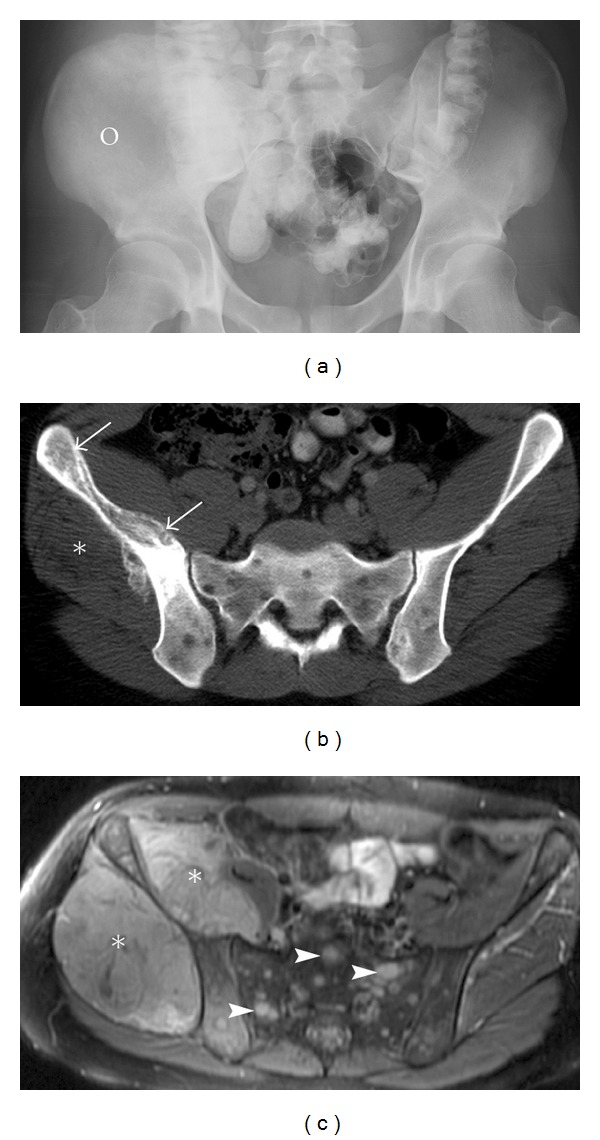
Ewing's sarcoma. A 21-year-old male with advanced Ewing's sarcoma. AP radiograph (a) of the pelvis reveals a vague mixed density abnormality diffusely involving the right ilium (circle). Note the asymmetry when compared to the normal left ilium. Incidental note is made of oral contrast in the bowel. CT scan (b) of the pelvis demonstrates permeative lesion of the right ilium (arrows) and an associated soft tissue mass (asterisk). Small multifocal lytic lesions in the sacrum and bilateral ilium are in keeping with metastasis. Axial T2 image (c) of the pelvis reveals a large exophytic soft tissue mass (asterisk) along the anterior and posterior borders of ilium. Note the predominance of a huge soft tissue mass when compared to the bone abnormality, characteristic of small round blue cell tumors. Marrow edema is noted in the affected right ilium and multiple T2 bright lesions (arrowheads) in the sacrum and right ilium represent metastasis.
